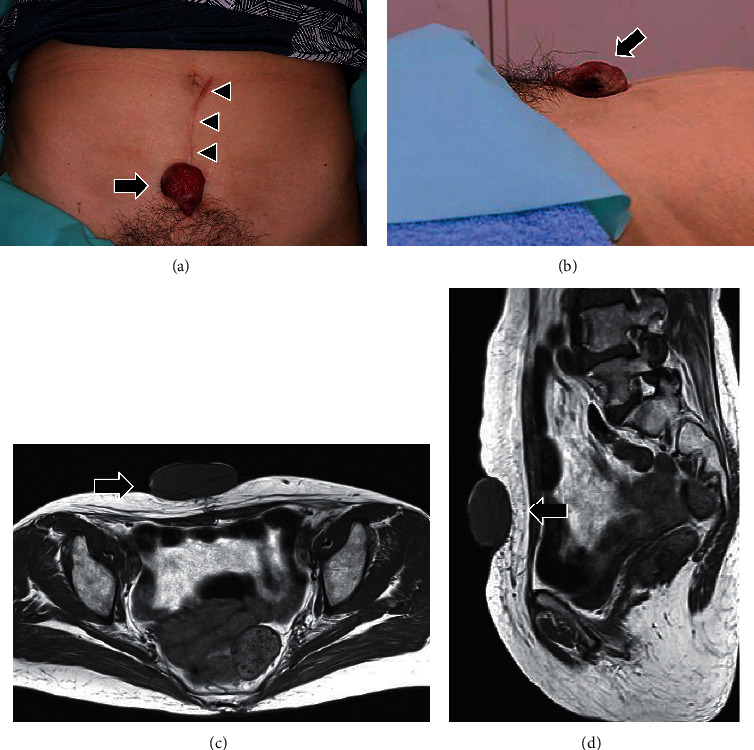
Frontal (a) and lateral (b) view of the large protruding tumor (arrow) located on the abdominal wall. The lesion measured 3 × 3 × 2 cm at the first visit. A keloid scar (arrowhead) is visible on the abdominal wall due to partial resection of endometrial cancer. Axial (c) and sagittal (d) view of magnetic resonance imaging revealing a tumor protruding subcutaneously with no continuity to the abdominal cavity (arrow).
